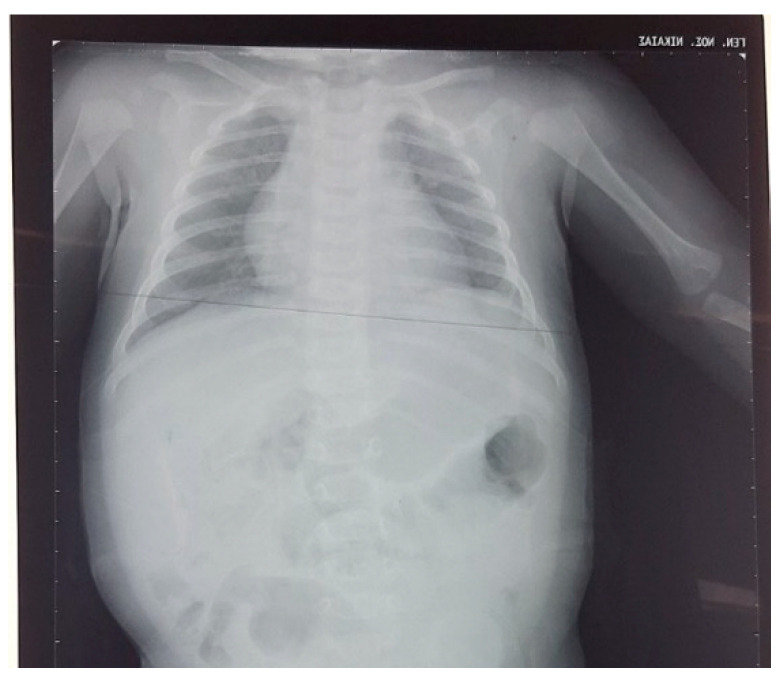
Chest radiograph at the age of 3 months.
